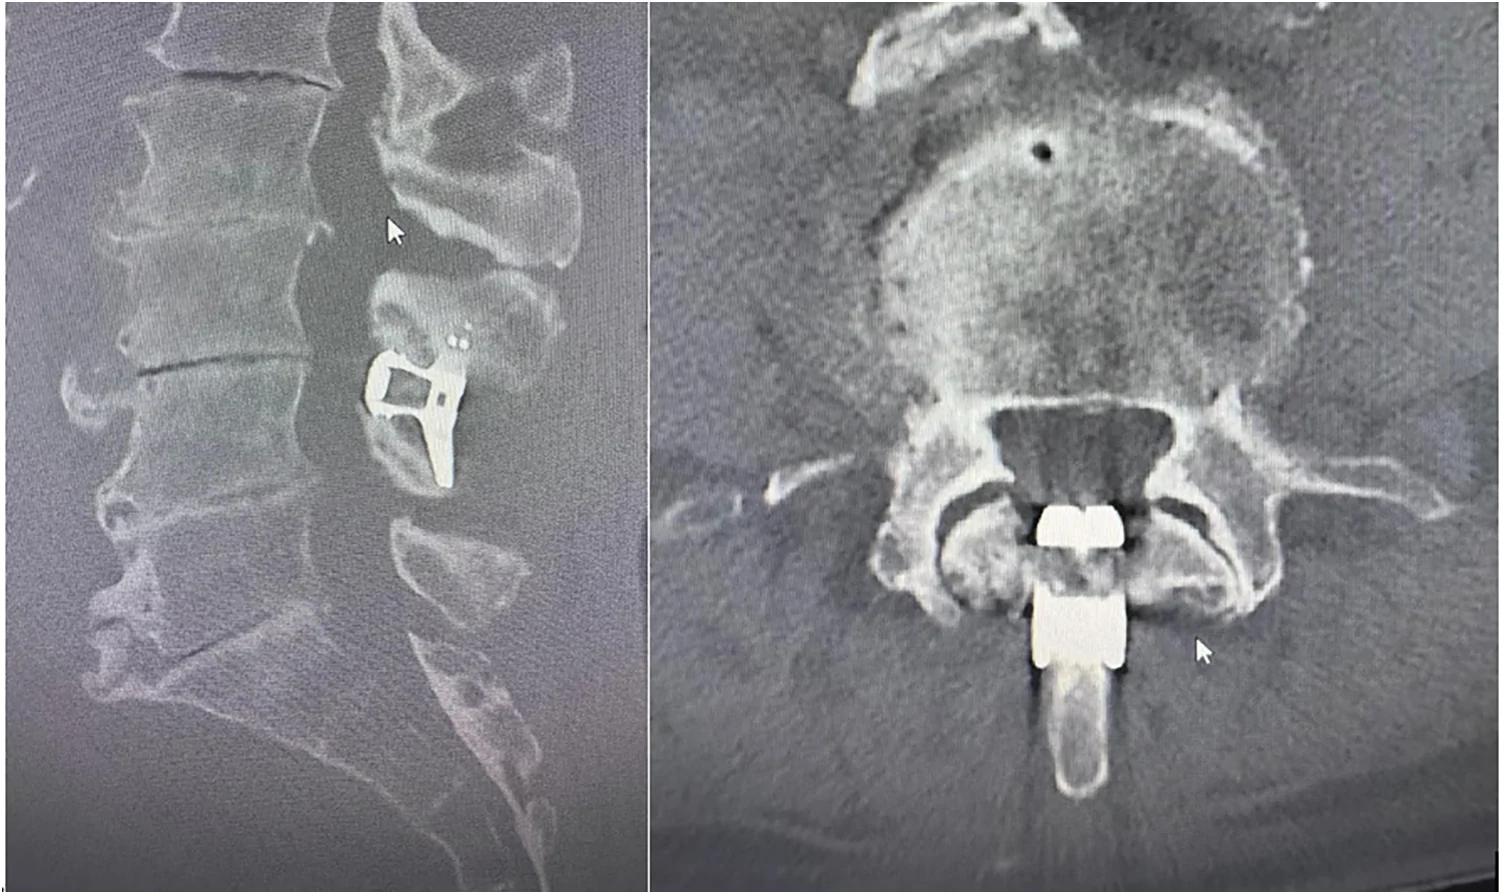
Computed tomography (CT) images demonstrating successful placement of novel interlaminar device.
